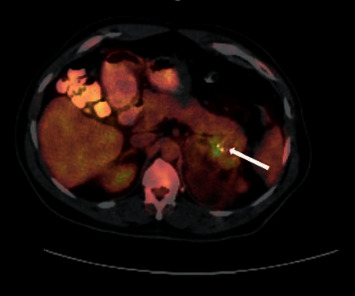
A positron emission tomography (PET) scan demonstrating metabolic uptake with focality localizing to the enhancing solid component of the left adrenal mass seen on the multiphase CT study.
